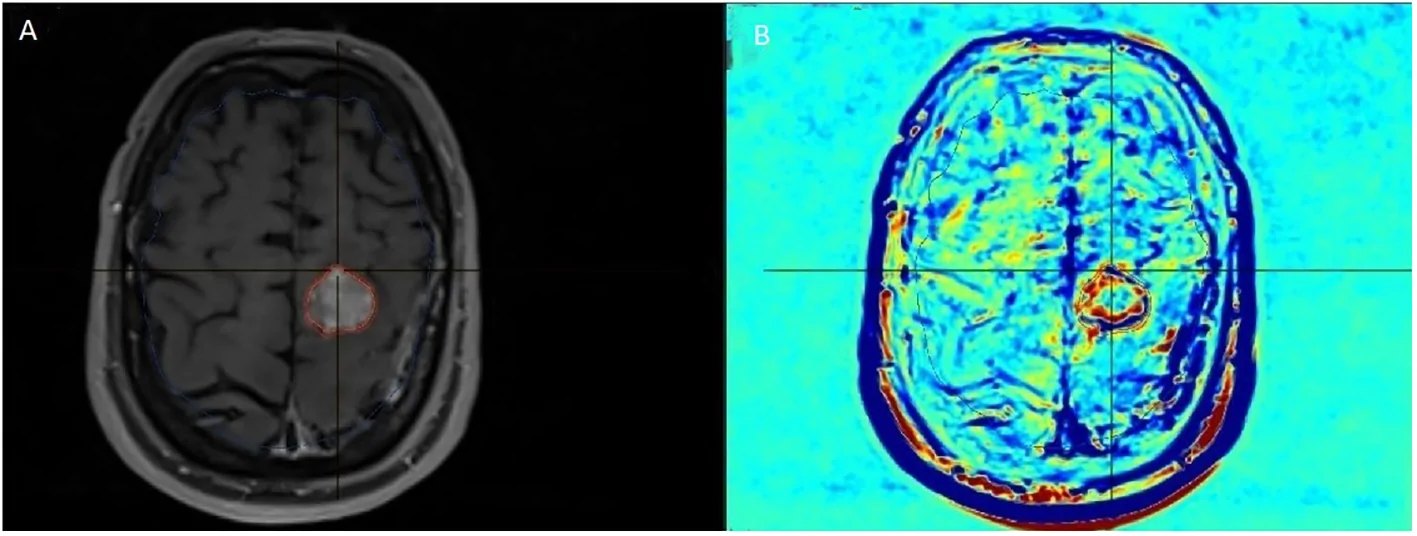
Post-operative TRAMs/CCA findings demonstrating a thin peripheral rim of contrast clearance adjacent to areas of delayed contrast retention surrounding the resection cavity. (A) Contrast-enhanced T1-weighted MRI performed 28 days after resection for SRS planning demonstrating heterogeneous signal intensity within the resection cavity and equivocal marginal enhancement. (B) Corresponding TRAMs/CCA demonstrating a central area without significant contrast clearance or delayed contrast retention, surrounded by delayed contrast retention and a thin peripheral rim of early contrast clearance.
